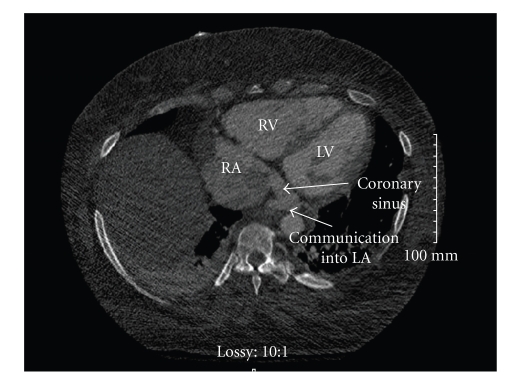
Cardiac CTA. The arrows indicate the coronary sinus with a communicating vessel to the LA.
